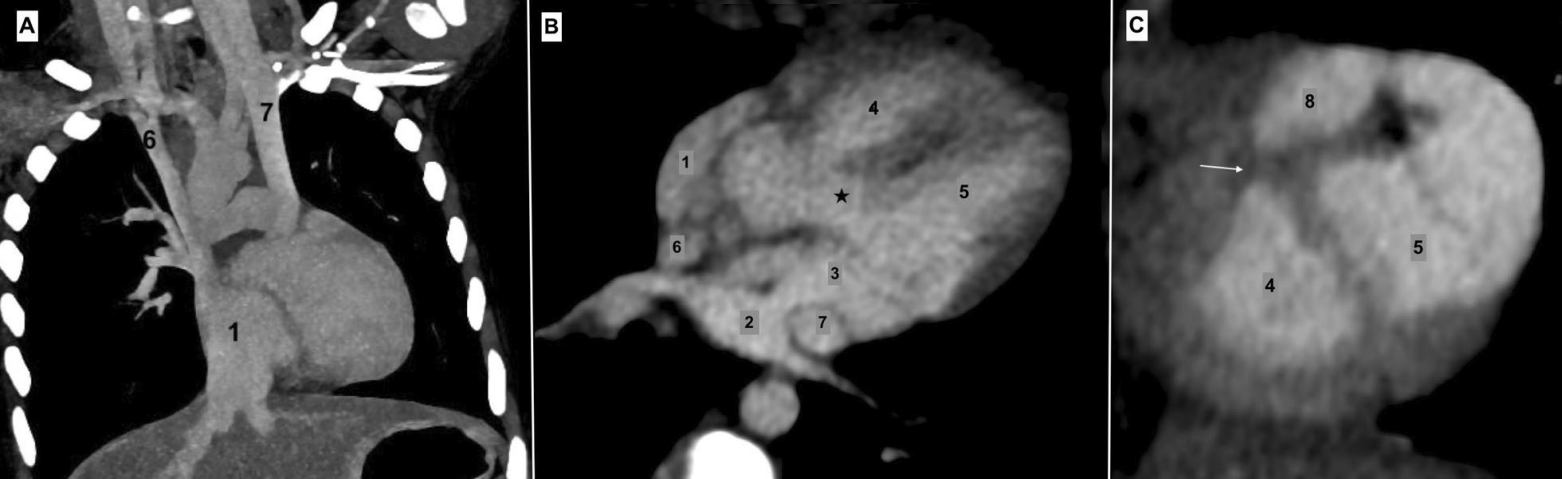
CT Angiography. A Coronal section showing double SVC draining into RA. B Four-chamber view showing cor triatriatum, VSD (*) and double SVC. C Two-chamber view showing the infundibular chamber and the hypertrophied obstructing muscle bands (arrow) dividing the RV into two chambers. 1—Right atrium, 2—posterior chamber of left atrium, 3—anterior chamber of left atrium, 4—right ventricle, 5—left ventricle, 6—right superior vena cava, 7—left superior vena cava, 8—infundibular chamber
